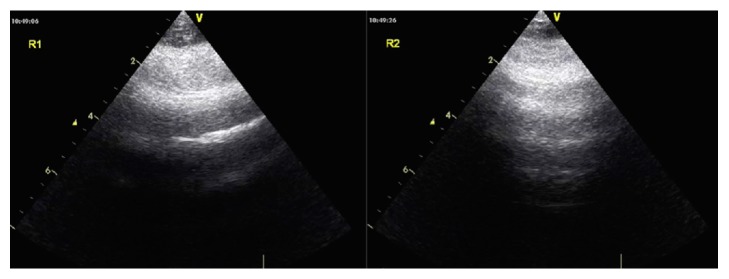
Part of lung ultrasound in the repeated ultrasound exam in the next day. Bilateral A-lines in bilateral 1st regions indicate pulmonary edema was obviously decreased.
